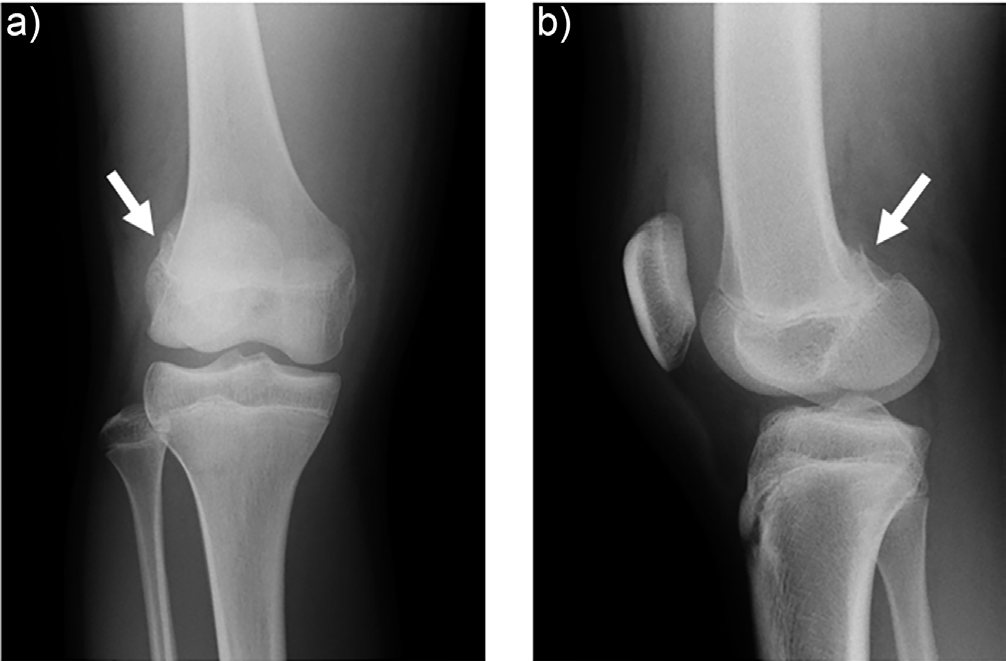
Anteroposterior a) and lateral b) radiograph show the injured knee with a minimal fracture of the lateral femoral condyle (arrow).
